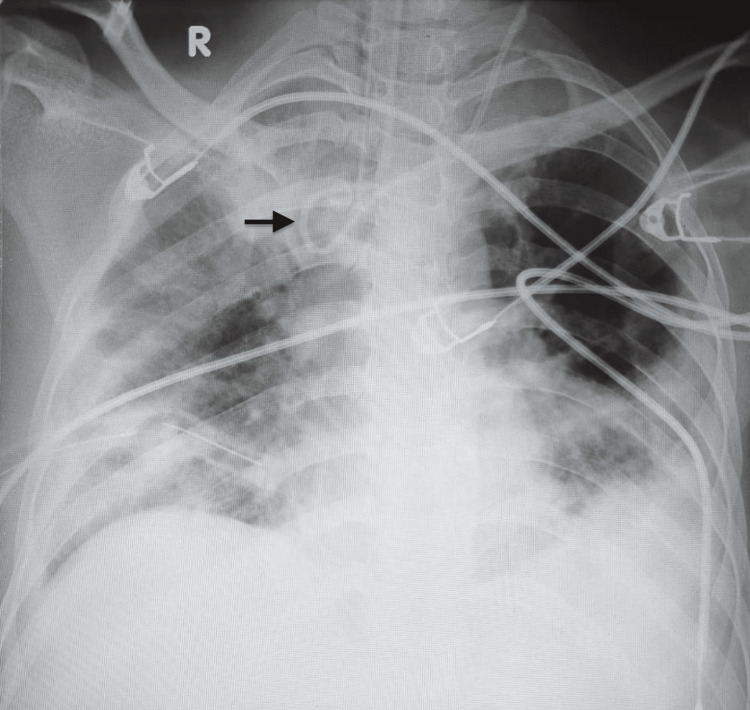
Postoperative chest X-ray showing coiled left internal jugular venous catheter tip (black arrow) forming a fishhook pattern.
